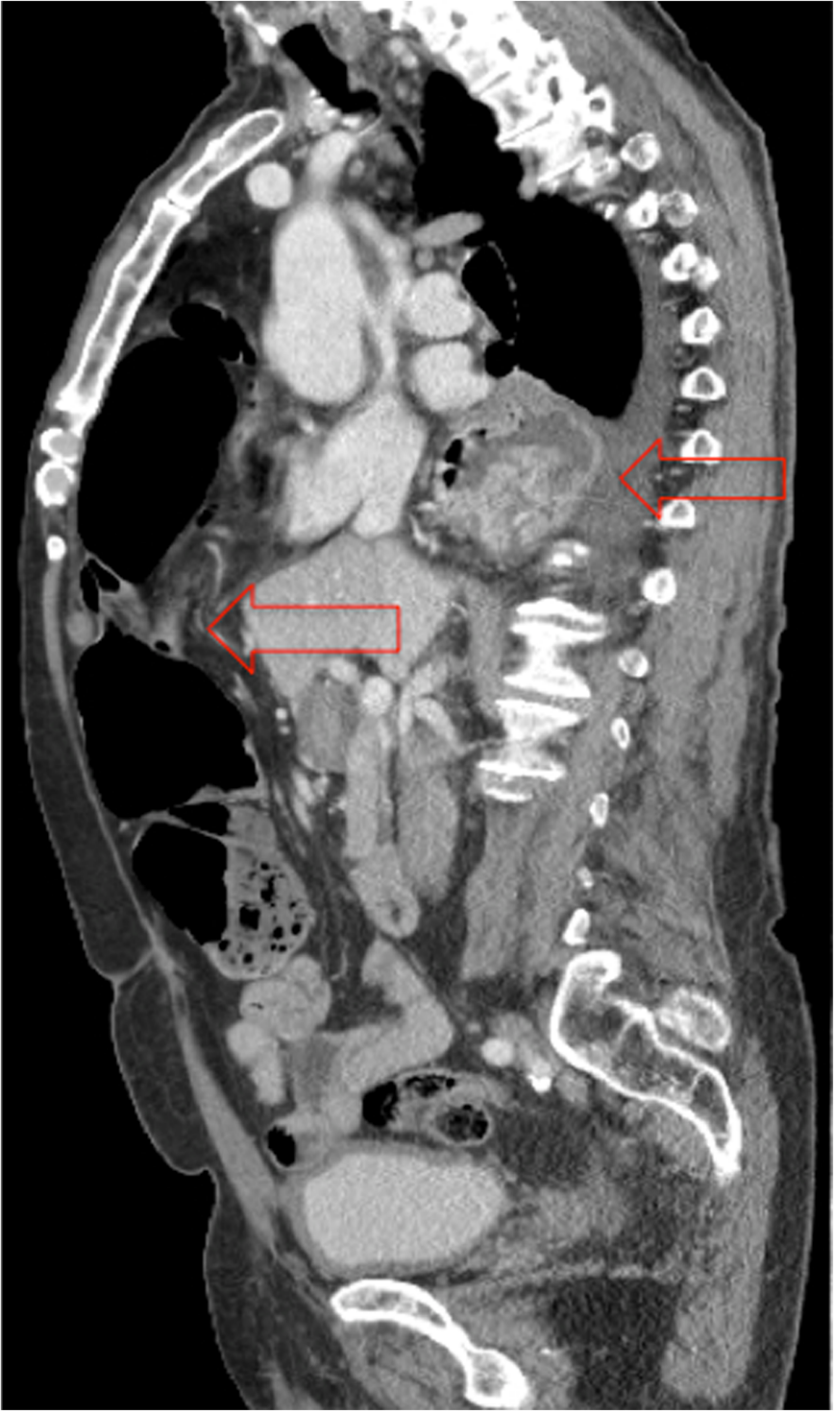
Computed tomography scan obtained 8/2013, sagittal view with arrows showing anterior Morgagni defect and posterior paraesophageal hernia. Computed tomography scan obtained 8/2013, sagittal view with arrows showing anterior Morgagni defect and posterior paraesophageal hernia.
